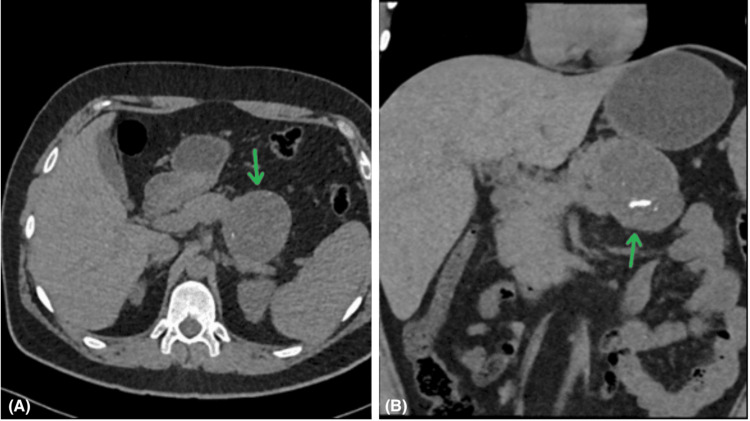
Axial (A) and coronal (B) plane CT scan showing a well-defined low attenuation mass centered in the pancreatic tail region with intralesional speckles of calcification (green arrows).
